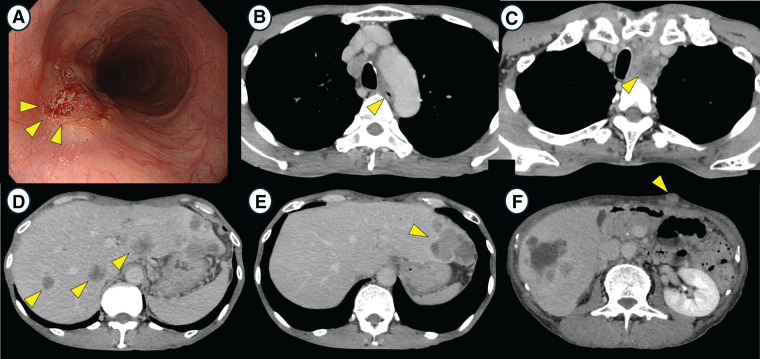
Imaging findings at the time of initial diagnosis (A) Upper gastrointestinal endoscopy showing a Borrmann type 3 tumor in the middle thoracic esophagus (arrows). Biopsy confirmed moderately differentiated SCC. (B) Contrast-enhanced CT (CECT) indicating the location of the esophageal tumor (arrow). (C) An enlarged 106recL lymph node (arrow), suggesting lymph node metastasis. (D) Multiple liver metastases (arrows). (E) A metastatic lesion in the left lateral segment of the liver (arrow). (F) A subcutaneous nodule in the abdomen, which was pathologically diagnosed as a cutaneous metastasis of poorly differentiated SCC (arrow). SCC, squamous cell carcinoma
